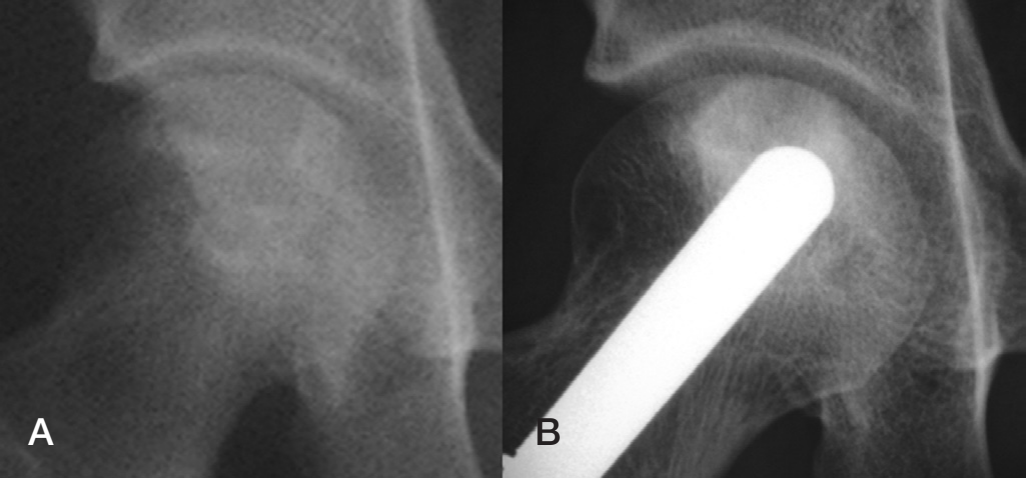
Right hip of a 15-year-old girl with osteonecrosis of the femoral head secondary to cortisone intake for marrow transplantation. A. Preoperatively, Steinberg stage II with HHS of 85. B. 5 years later, radiographic Steinberg staging remained unchanged and HHS was 100.
